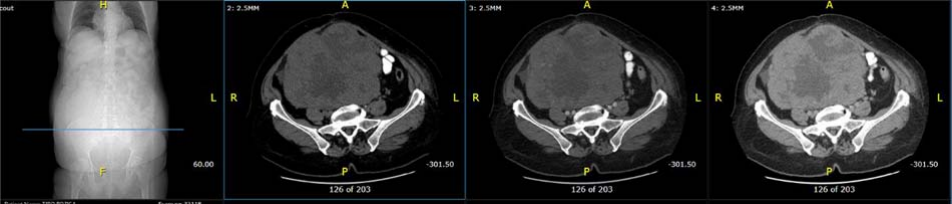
Axial computed tomography (CT) scan shows solid mass in abdominopelvic cavity.
